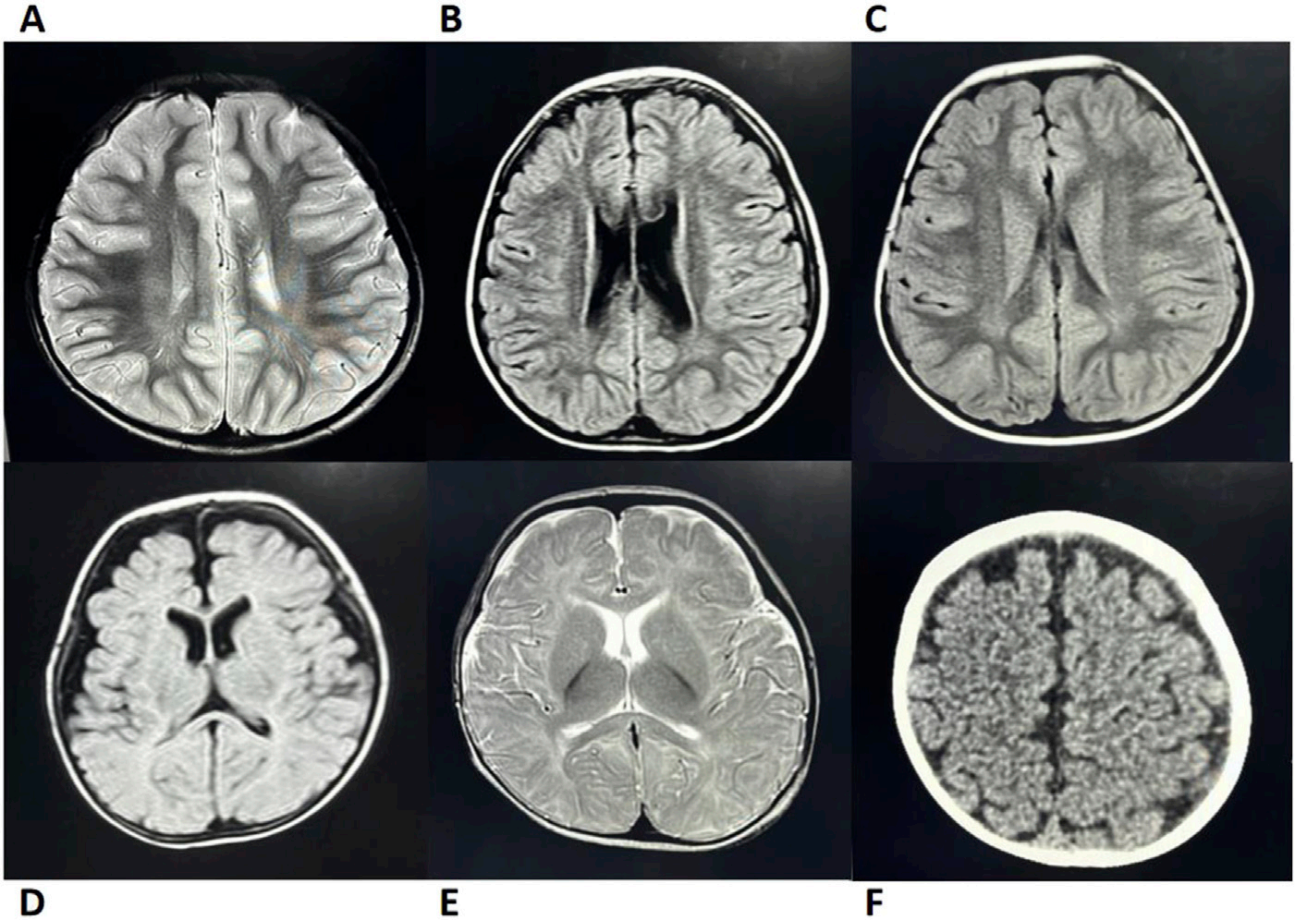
Brain MRI/CT Imaging of Cases 2, 5, 6, 9, 12, and 14 (A) Case 5; (B) Case 9; (C) Case 14; (D) Case 2; (E) Case 12; (F) Case 6. Case 5: MRI revealed patchy T2 hyperintensities adjacent to the posterior bilateral lateral ventricles. Cases 9 and 14: Both exhibited patchy FLAIR hyperintensities near the posterior bilateral ventricles (Note: Abnormal MRI findings in Case 14 resolved with age, while only Case 9 presented with delayed language and social development). Case 2: MRI showed bilateral frontotemporal subdural FLAIR hyperintensities, suggesting subdural effusion. Case 12: MRI demonstrated T2 hyperintensity in the genu of the corpus callosum with delayed myelination at 8 months of age (consistent with clinically observed psychomotor retardation). Case 6: CT imaging demonstrated widened cerebrospinal fluid spaces. Imaging Modality: All cases underwent MRI except Case 6 (CT); T2-weighted abnormalities in Cases 5/12 and FLAIR changes in Cases 9, 14, and 2. CT, computed tomography; MRI, magnetic resonance imaging.
